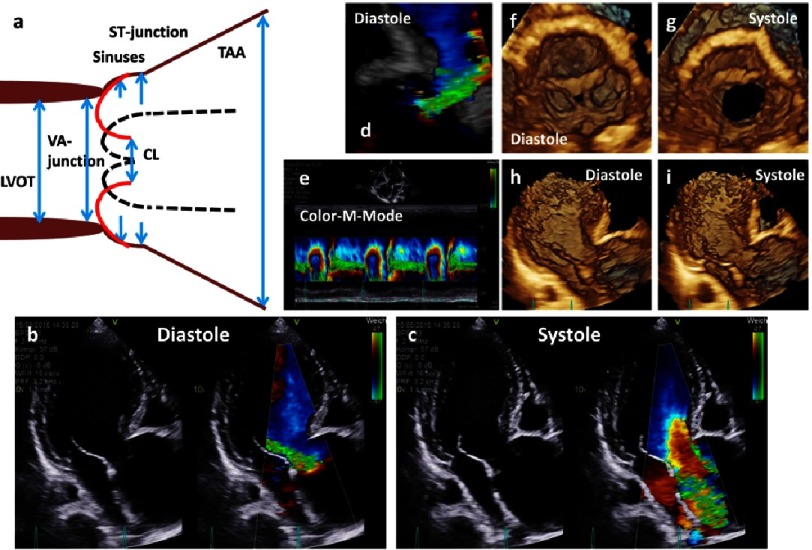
The scheme shows an ectasia of the sinuses of Valsalva and a severe aneurysm of the proximal TAA (a). Below native and color-coded 2D transthoracic images are shown during diastole (b) and systole (c). In addition, 3D TTE image of the aortic regurgitation during diastole (d), color M-Mode of the regurgitation (e), en-face views of the aortic annulus during diastole (f) and systole (g) and 3D transthoracic images of long axis views during diastole (h) and systole (i) are shown.
